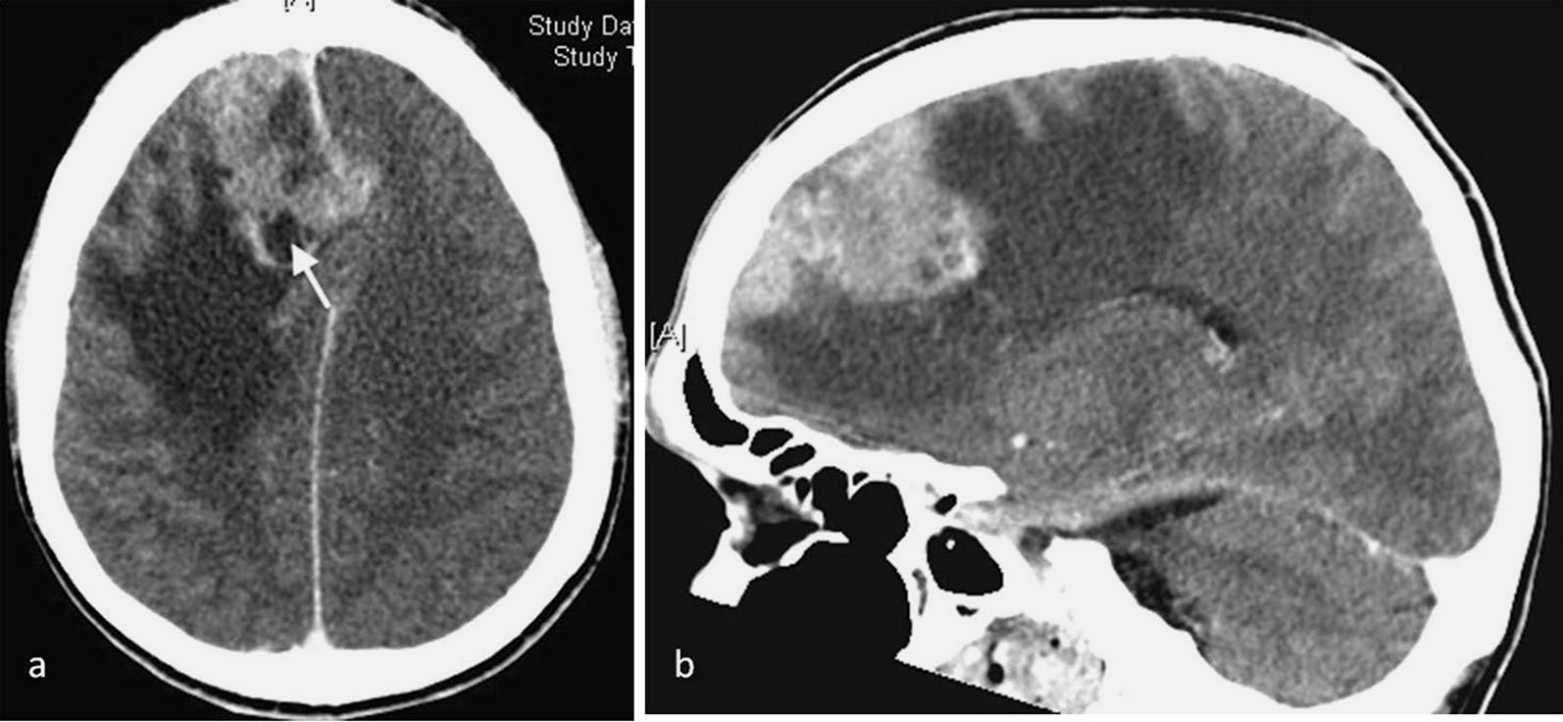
Axial CT post contrast image (a) and sagittal reconstruction (b) shows an irregular extra-axial solid mass with heterogeneous enhancement and deep cystic change (*arrow*) adjacent to the falx extending into the right frontal lobe. There is significant peritumoral oedema and mass effect involving the right frontal lobe with displacement of the midline. These findings, together with tumour interdigitating with the brain substance, are consistent with an aggressive frontal meningioma
